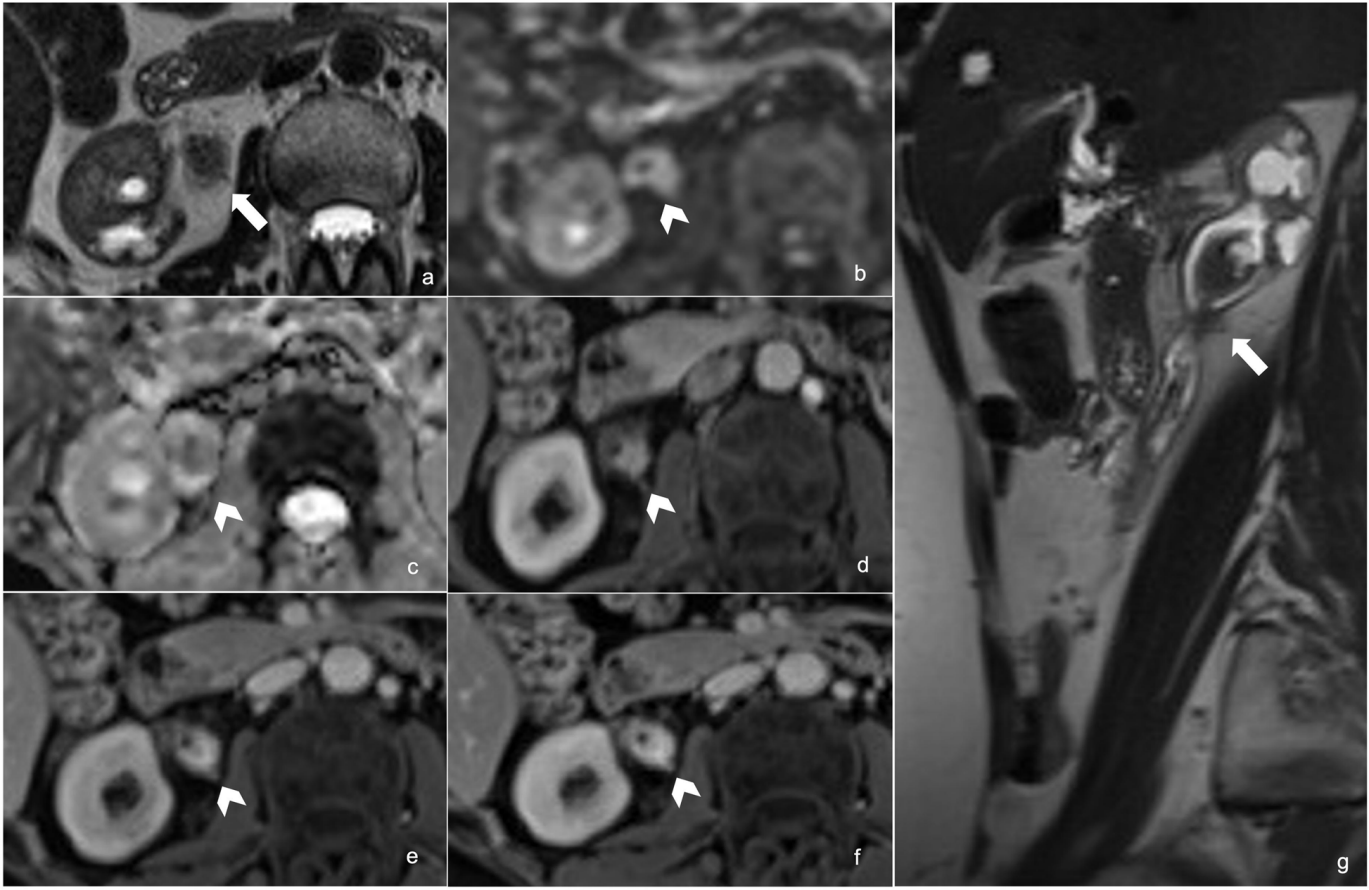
A 64-year-old female patient presenting with gross hematuria and neoplastic cells at urine cytology. a, g T2WI (axial and sagittal plane) shows an endophytic lesion (13 × 20 mm) of the right pyelo-ureteral junction (white arrow), which determines stenosis of the lumen, third-grade hydronephrosis on the same side and adjacent “fat stranding” appearance. b, c DWI (*b* = 1,000 s/mm^2^) and ADC map show a lesion with a significant restriction of diffusion (white arrowhead). d, e, f Dynamic sequences after gadolinium-based contrast injection (corticomedullary, nephrographic, and excretory phase) show an early lesion enhancement, extending through the muscularis layer (white arrowhead). All these MRI findings are suspicious of muscle invasion. At final pathology, the lesion proved to be muscle-invasive. *ADC*, Apparent diffusion coefficient; *DWI*, Diffusion-weighted imaging; *T2WI*, T2-weighted imaging
